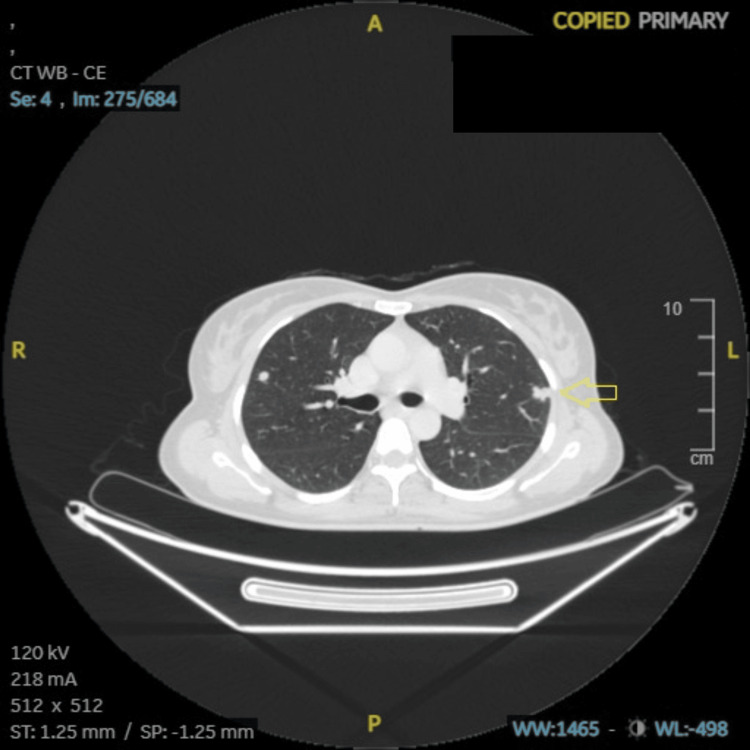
Case 2: CECT (abdomen and pelvis), soft tissue nodules in left lung upper lobe (6 × 4.5 cm), multiple soft tissue nodules in bilateral lung parenchyma (arrow). CECT: contrast-enhanced computed tomography, A: anterior, R: right, L: left, P: posterior, ST: soft tissue, SP: soft tissue window (preset), WB: whole body, WW: window width, WL: window level.
